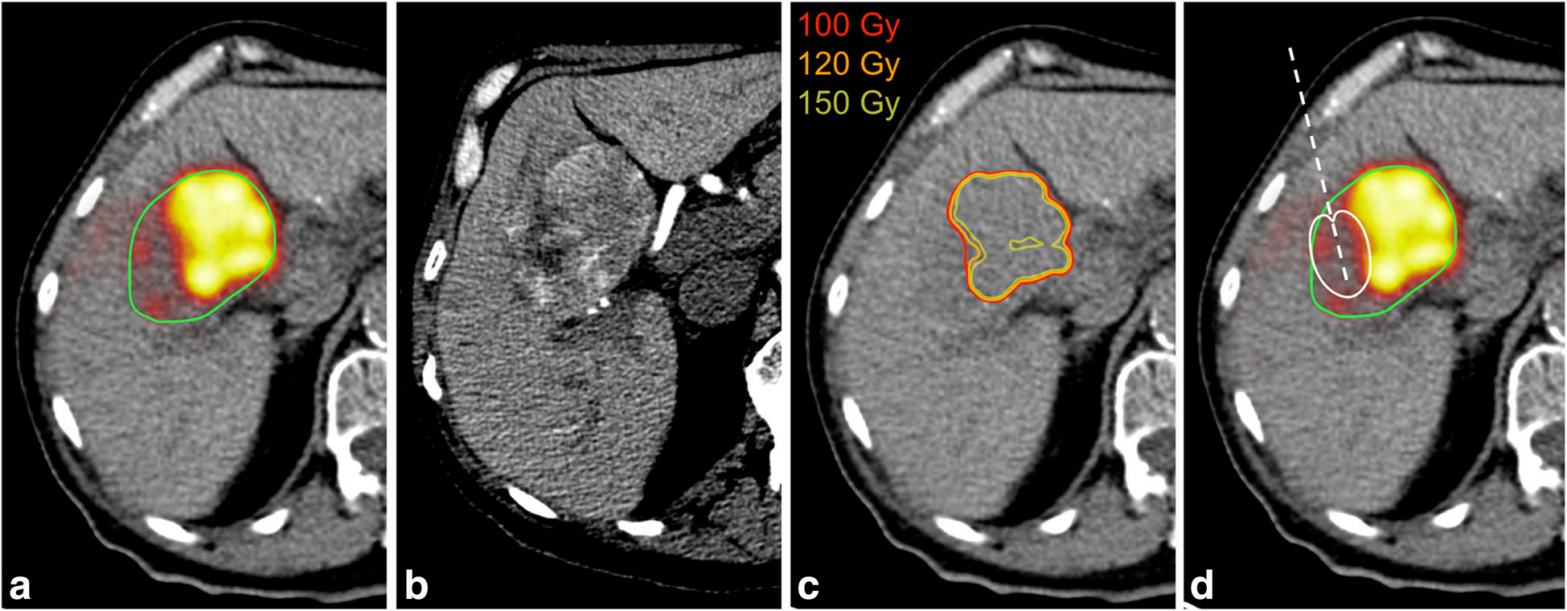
^90^Y PET/CT-guided treatment plan for patient 1 (Table 1). a Post-radioembolization ^90^Y PET/CT with tumor contour (*green*). b Pre-treatment hepatic protocol CT. c 100, 120, and 150 Gy isodose curves computed from post-radioembolization ^90^Y PET/CT using the local deposition method. d Electrode placement (*dashed white line*) and RF ablation cell killing boundary (*solid white line*, Ω = 6.9)
